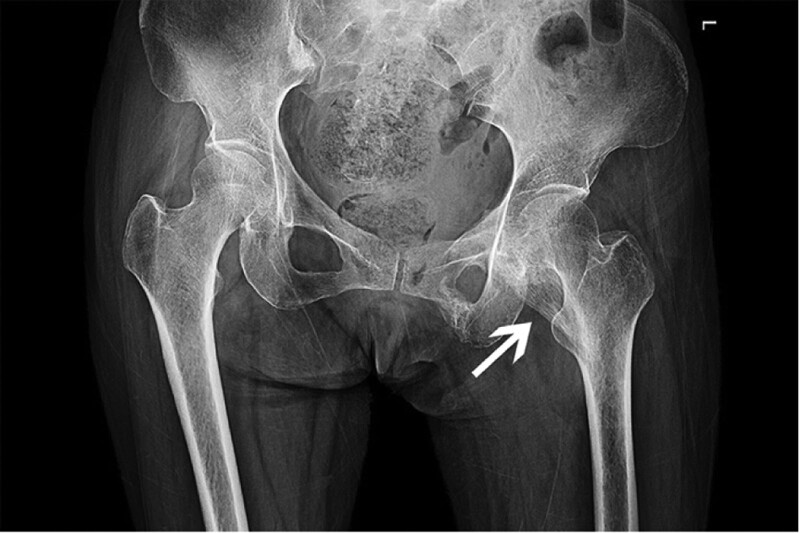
Radiograph of the pelvis taken 4 mo after the accident reveals an abnormal lesion with calcified radial fibers extending from the superior pubic ramus to the lesser trochanter (white arrow).
